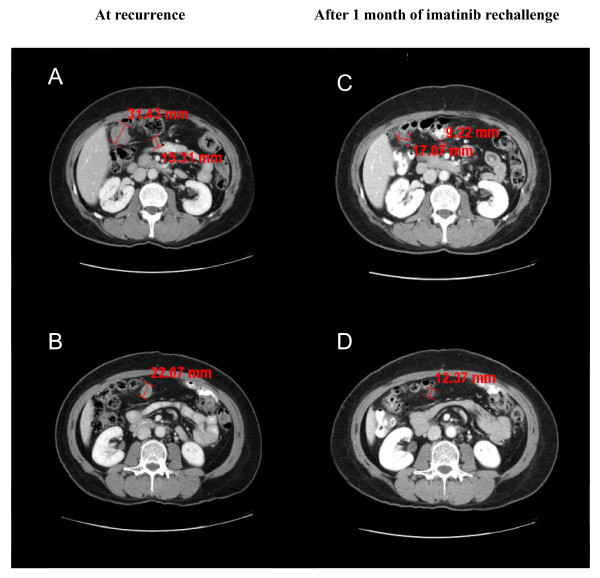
CT scans of our patient before and after imatinib rechallenge. (A,B) Recurrent tumors (nodules) can be seen in the peritoneum of our patient, who had received prior adjuvant imatinib treatment. (C,D) After one month of rechallenge with imatinib, our patient demonstrated a partial response to imatinib treatment, with the nodule sizes decreasing by 43%.
